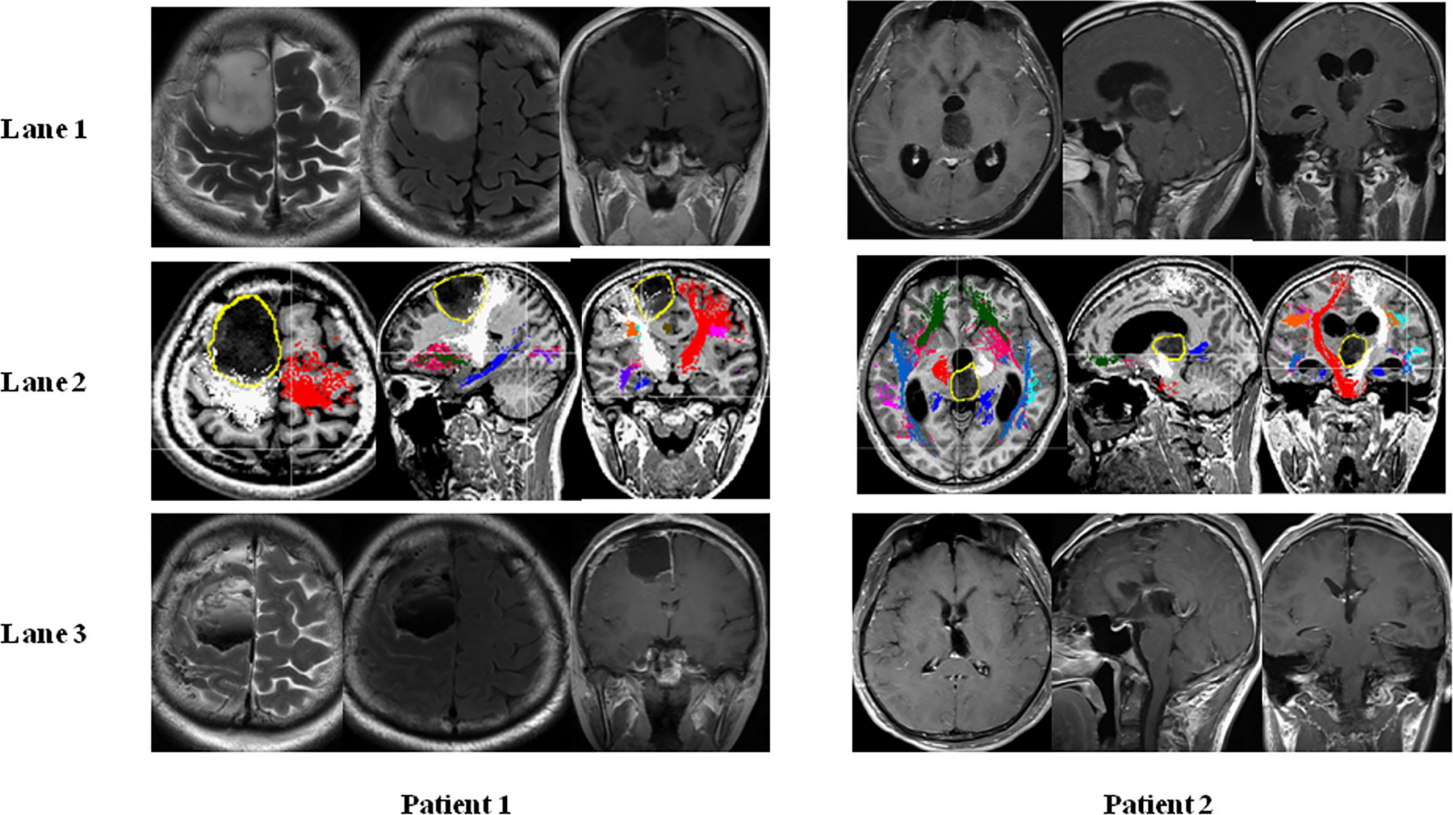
Minimal subcortical monopolar threshold (MSCMT) and the proximity to the motor pathway in patient 1 (astrocytoma in the supplement area with MSCMT = 3.4 mA and motor deficit after surgery) and patient 2 (anaplastic astrocytoma in the thalamus with MSCMT = 5.6 mA and no motor deficit after surgery). MR images are shown in lane 1 (before surgery), lane 2 [the proximity of tumor with the pyramid tract in the diffusion tensor imaging (DTI)], and lane 3 (after surgery).
